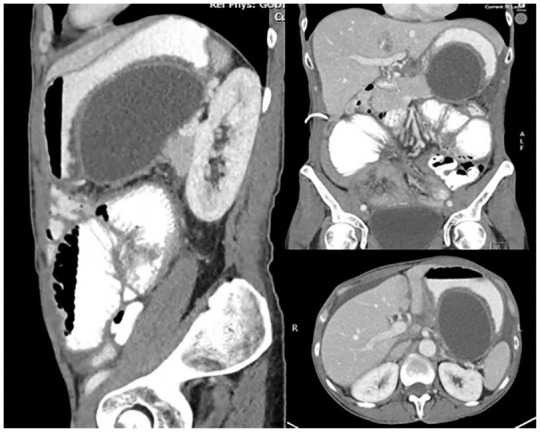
Sagittal, coronal and axial CT images of the large loculated malignant ascites at the posterior aspect of the stomach.
